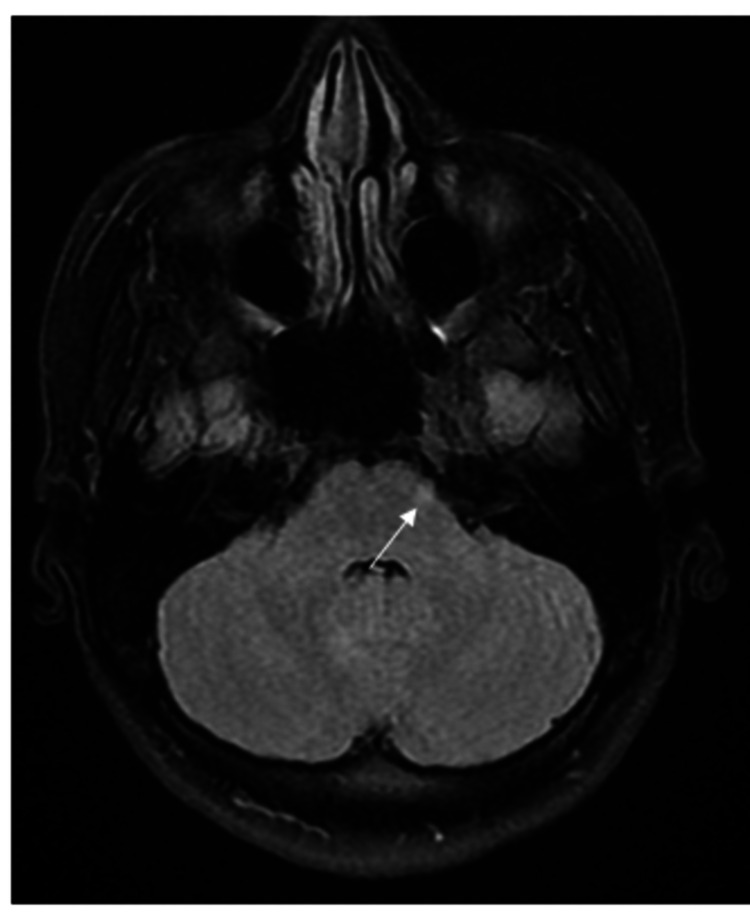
Brain MRI showing hyperintense FLAIR lesion in the rostral lateral pontine area (arrow) MRI: magnetic resonance imaging; FLAIR: fluid-attenuated inversion recovery
